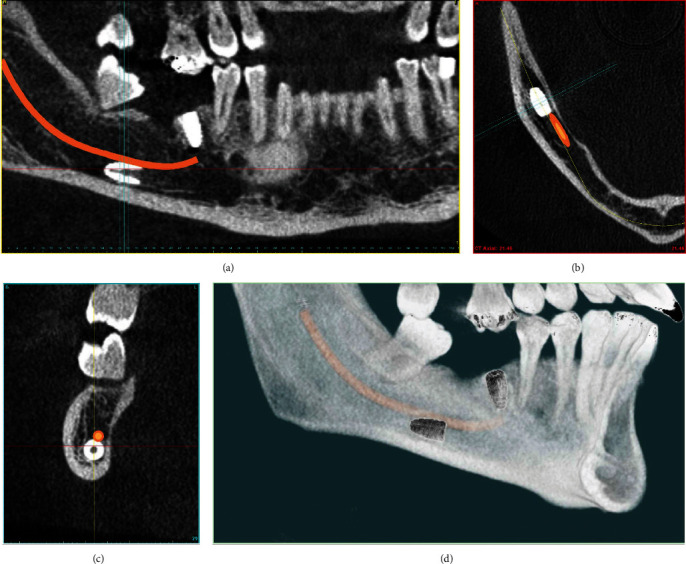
CBCT after implant dislocation. (a) Reformatted panoramic image. (b) Axial reconstruction. (c) Sagittal reconstruction. (d) 3D reconstruction of the mandible (lingual view). All images show that the implant is located below the level of the mandibular canal.
